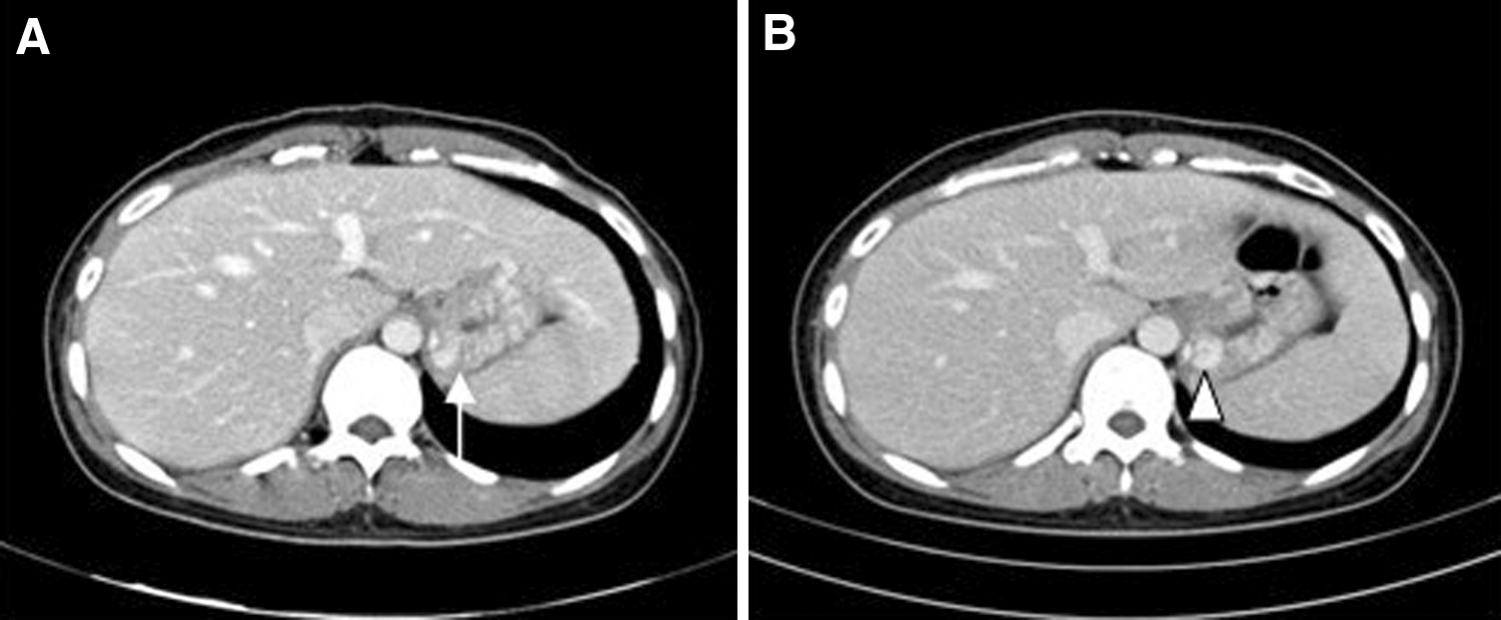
A A patient with grade 2 perigastric varices following lap-WT revealed at the 12-month follow-up by CT scan (*white arrow*). B At 4 years postoperatively, perigastric varices above 10 mm were still evident in this patient, but there were no symptoms and no gastrointestinal bleeding (*white arrow head*)
